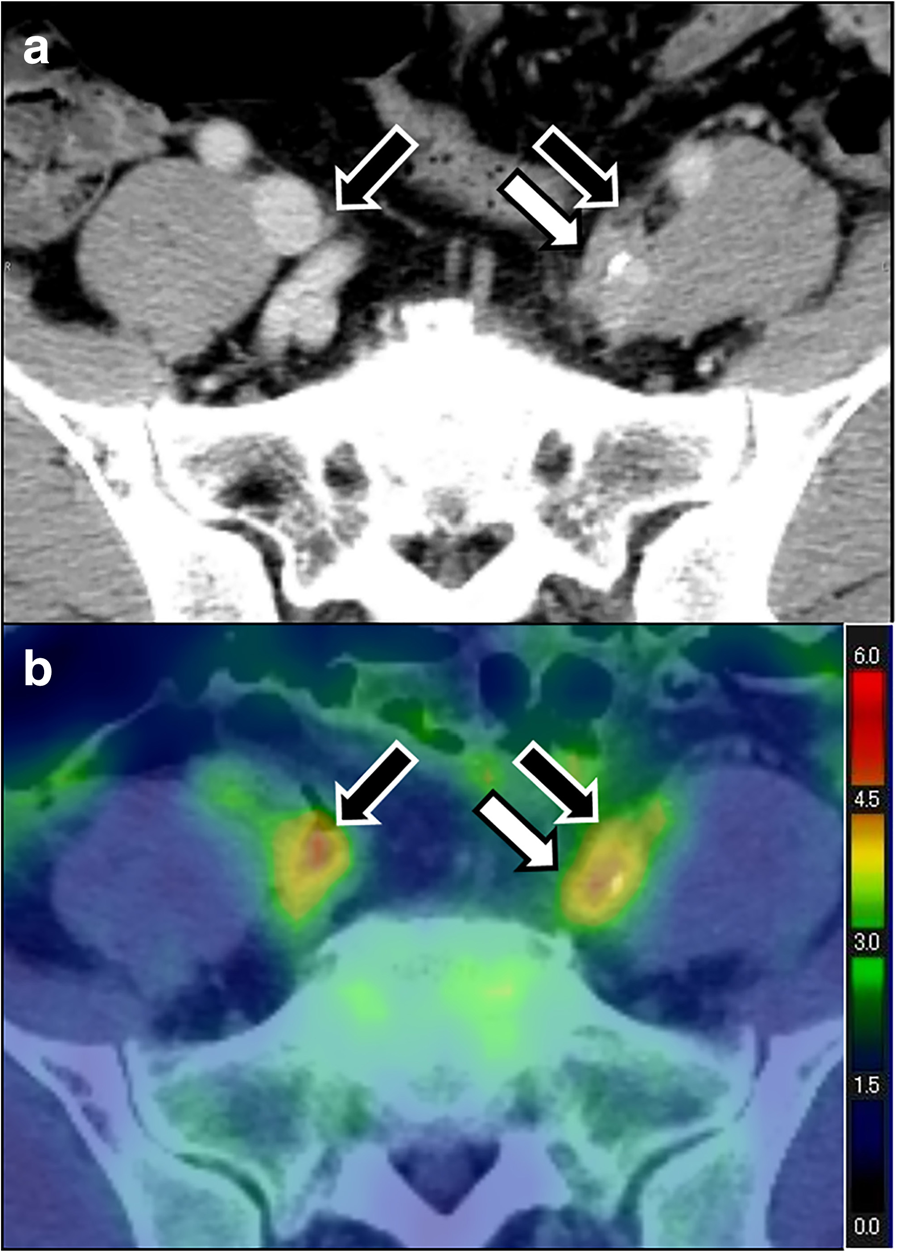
CECT (a) and FDG-PET/CT (b) images demonstrating left internal iliac artery involvement (*white arrow*). Physiological uptake by the urinary tracts (*black arrows*) cannot be differentiated using FDG-PET/CT alone
